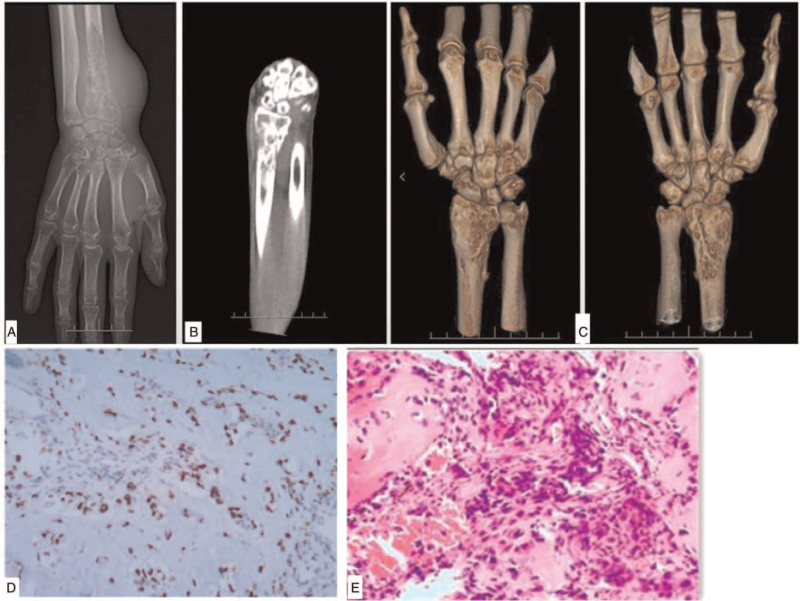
Preoperative examination. (A–C) The x-ray film and computed tomography scan. multiple osteolytic bone destruction occurred at the distal end of the right radius and the surface of wrist joint, with several discontinuous bone cortex. The distal bone was slightly enlarged, with slight periosteal reaction. d.e pathological biopsy. (D) Preoperative biology and (E) second biology at the surgery: osteogenic malignant tumor.
